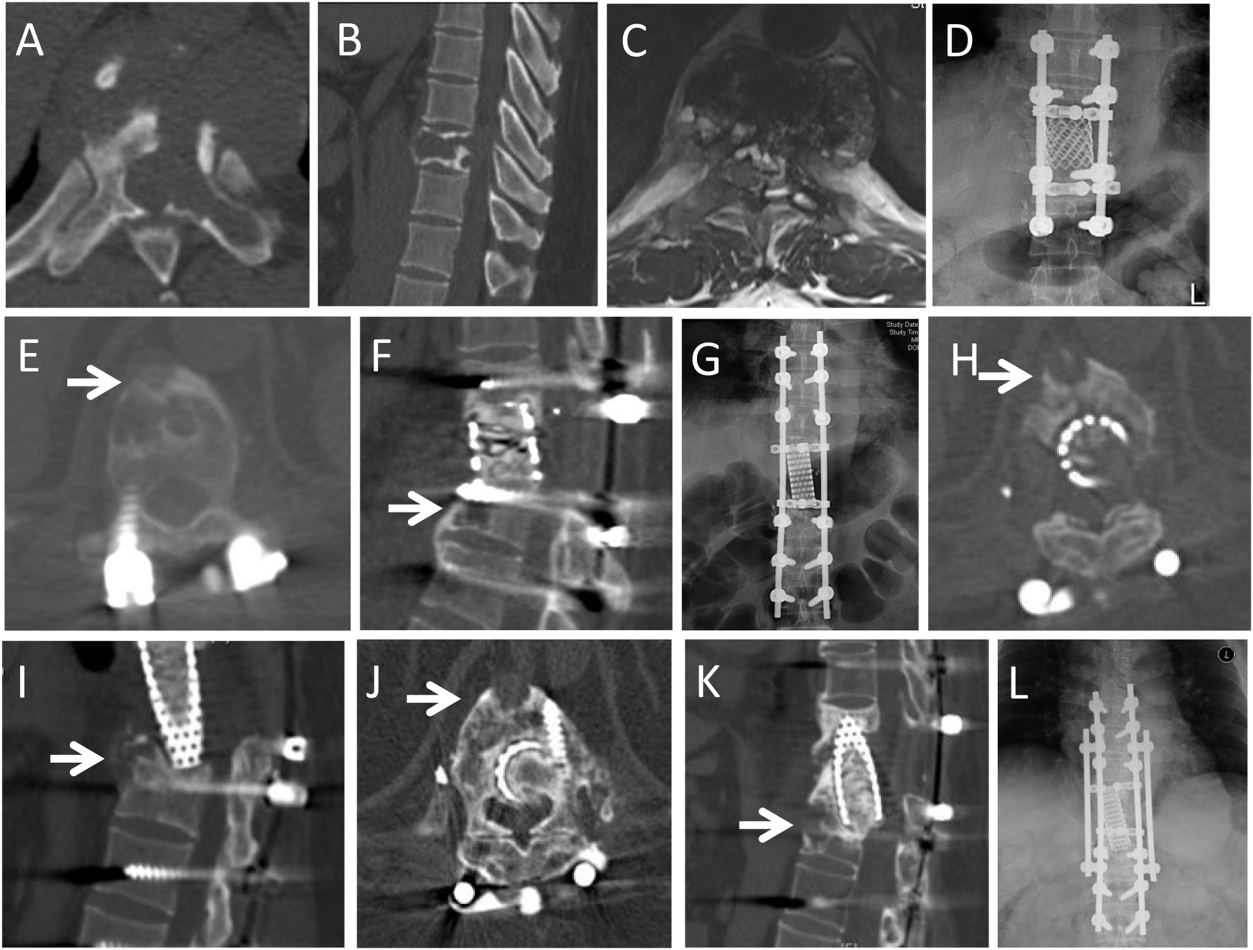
A 46-year-old male patient presented with back pain for one month. CT and MRI showed an osteolytic lesion in the T9 vertebra. He underwent GTR (en bloc vertebrectomy of T9). At 37-month follow-up, he complained of back pain for 3 months, and CT and MRI demonstrated local recurrence at T10 vertebral body (arrows in E,F). He underwent GTR (en bloc vertebrectomy of T10). At 19 months after second operation, he had a sudden back pain and X-ray showed that rod fracture. He underwent the third operation to replace the broken titanium rod with cobalt-chromium-molybdenum rod. At 8 months after third operation, the CT and MRI revealed the local recurrence at anterior part of T11 vertebral body (arrows in H,I). He underwent radiotherapy but refused further surgery. The recurrent lesion was stable in subsequent 52 months follow-up (arrows in J,K), but the rob fractured again. He had to underwent forth operation to replace the rod. (A,B): Preoperative axial and sagittal CT scans revealed the osteolytic lesion extended from the T9 vertebral body to the left pedicle and transverse process; (C): T2-weighted axial MRI scans; (D): Posteroanterior X-ray after first operation; (E,F): Axial and sagittal CT scans at 37-month follow-up after the first operation; (G): Posteroanterior X-ray after second operation; (H,I): Axial and sagittal CT scans 8 month later after third operation; (J,K): Axial and sagittal CT scans in 44th month after radiotherapy; (L): Posteroanterior X-ray after fourth operation.
